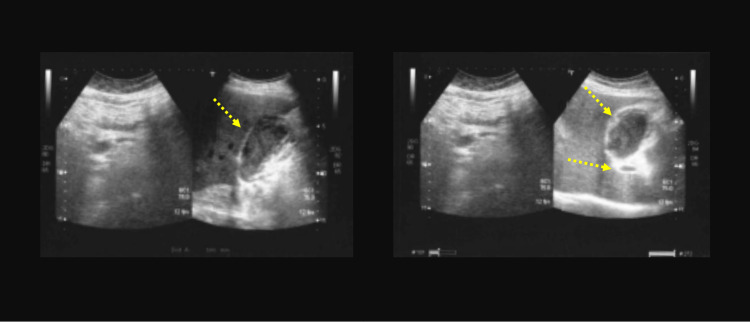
Preoperative ultrasound images mimicking acute lithiasic cholecystitis with atrophy and sclerosis. The longitudinal view of the gallbladder in the fasting state (left images, dotted arrow). The relationship of the gallbladder with the portal vein (right images, dotted arrows). The dilated loop of the bowel (right colonic flexure) is misinterpreted with acute lithiasic cholecystitis.
